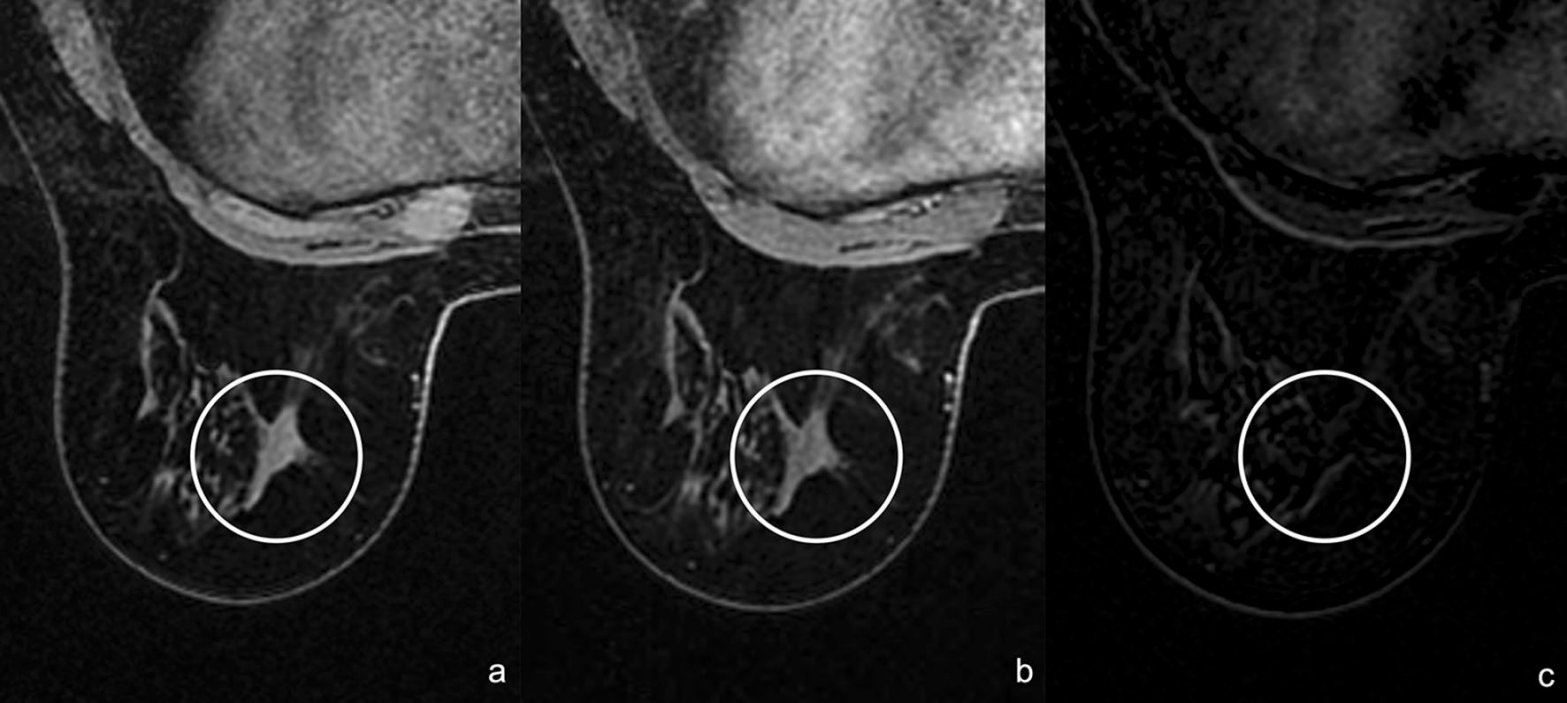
Axial MRI precontrast T1-weighted image (a), early contrast-enhanced T1-weighted image (b) and early T1-weighted subtraction show an architectural distortion without enhancement (white circle)
